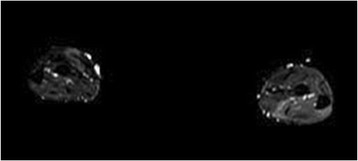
Bilateral forearm MRI post-exercise (symptomatic right arm, asymptomatic left arm)
